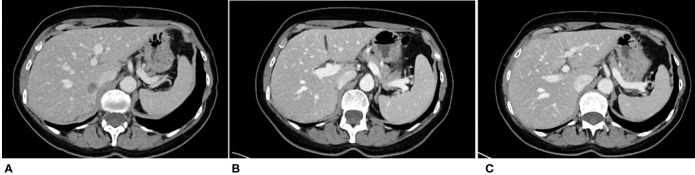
CT scans performed on January 2021 [(A), baseline poziotinib] and subsequently on March 2021 (B) and October 2021 (C) demonstrated a PR (B) and a disappearance of the lesion (C).
